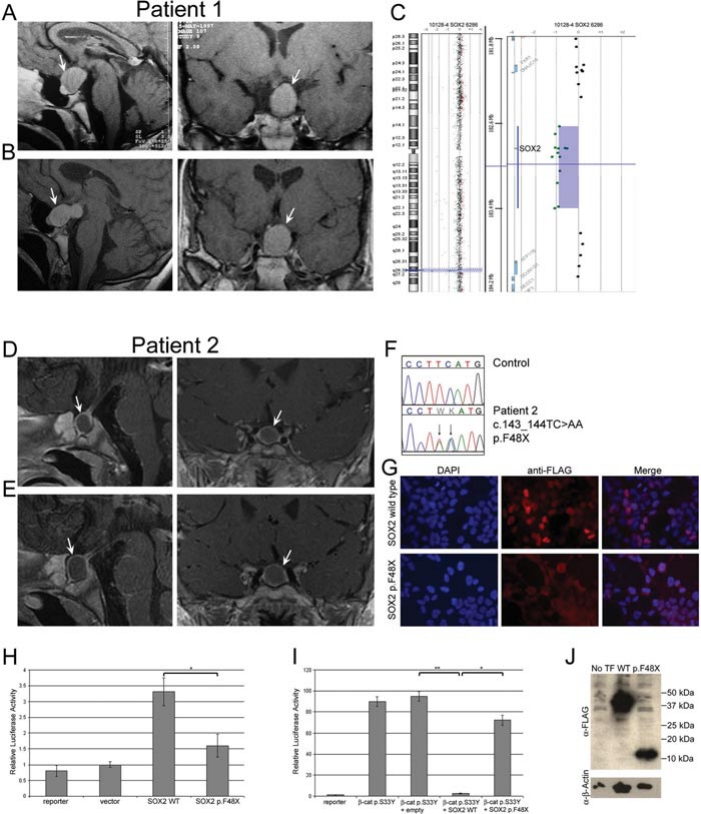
Identification and characterization of novel *SOX2* heterozygous mutations in association with slow-growing sellar tumors. (A and B) Midline sagittal (left) and coronal (right) MRI scans of patient 1 at 18 years (A) and 28 years of age (B) revealing a large mass in the location of the pituitary gland (arrows). (C) Array-CGH profile from patient 1 showing the ratio of probes, each represented by a single dot plotted as a function of chromosome position; loss of copy number of a probe shifts the ratio to the left showing deletion of *SOX2*. (D and E) Midline sagittal (left) and coronal (right) MRI scans of patient 2 at 17 months (D) and at 32 months of age (E) showing a large mass in the location of the pituitary gland (arrows). (F) Sequence electropherogram showing a two-base substitution c.143_144TT>AA (p.F48X) in patient 2. Nucleotide numbering reflects cDNA with +1 corresponding to the A of the ATG translation initiation codon in the reference sequence. (G) Overexpression of FLAG-tagged SOX2 p.F48X in HEK293T cells results in impaired localization of the protein whereas FLAG-tagged wild type SOX2 localizes in the nucleus. Nuclei are counterstained with DAPI. (H) Luciferase assay using the *Hesx1* minimal promoter demonstrates impaired activation of the promoter through expression of SOX2 p.F48X (*t*-test, *P* = 0.0006). (I) Luciferase assay using the TOPFLASH reporter reveals that expression of the SOX2 p.F48X mutant protein does not result in repression of Wnt signaling, activated by expression of a stable mutant form of β-catenin (p.S33Y) (*t*-test, *P* = 0.0002), whereas expression of wild-type SOX2 represses this activation (*t*-test, *P* < 0.0001). (J) Western blot analysis showing similar expression levels of both wild-type (WT) and p.F48X proteins. Although the intensity of the signal obtained with the α-FLAG antibody is not comparable, this is due to unequal loading of protein extracts in the gel, as shown by the loading control (α-β-Actin).
